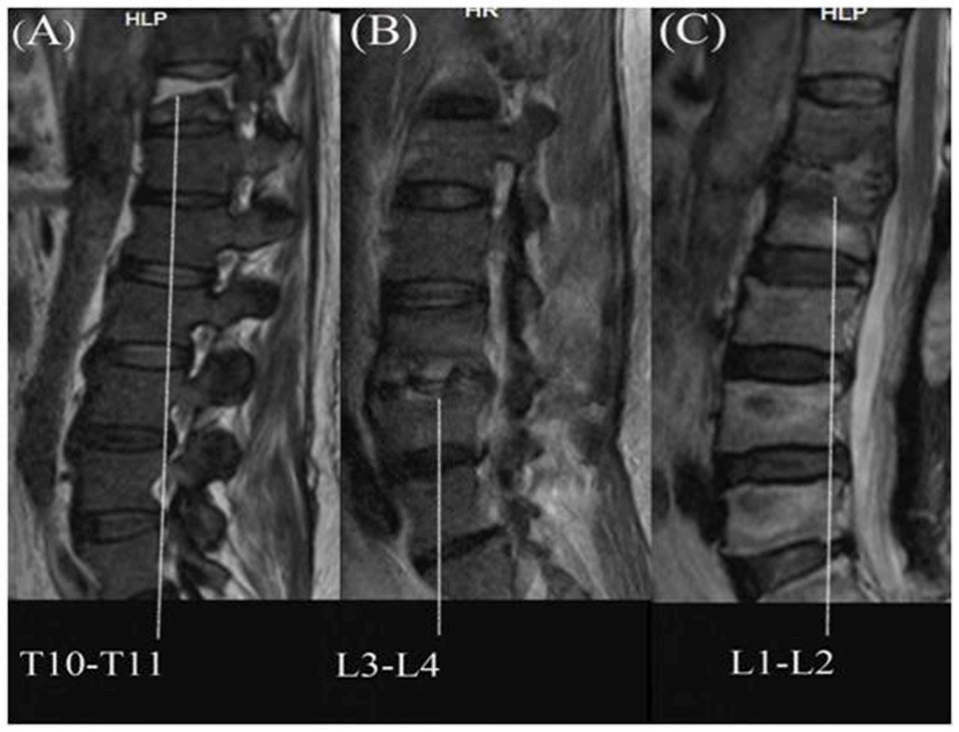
Magnetic resonance imaging of infectious spondylitis; (A) Tuberculous spondylitis; (B) Staphylococcal spondilitis; (C) NTM spondilitis.
